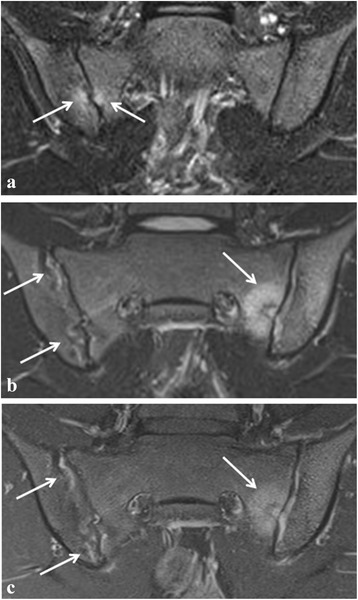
Active sacroiliitis in a 16-year-old girl with juvenile spondyloarthritis according to global assessment as well as to the ASAS definition of a positive MRI for sacroiliitis. a Semicoronal STIR image shows two small, focal spots of BME at the sacral and iliac side of the right sacroiliac joint (arrows). b Follow-up MRI 6 months later shows more extensive active sacroiliitis with bilateral high signal in the joint space and an active lesion with surrounding BME at the sacral side of the left sacroiliac joint (arrows). c Corresponding semicoronal fat-saturated T1-weighted image of the follow-up MRI shows bilateral enhancement of the synovium (synovitis) and of the active lesion on the left side (arrows)
